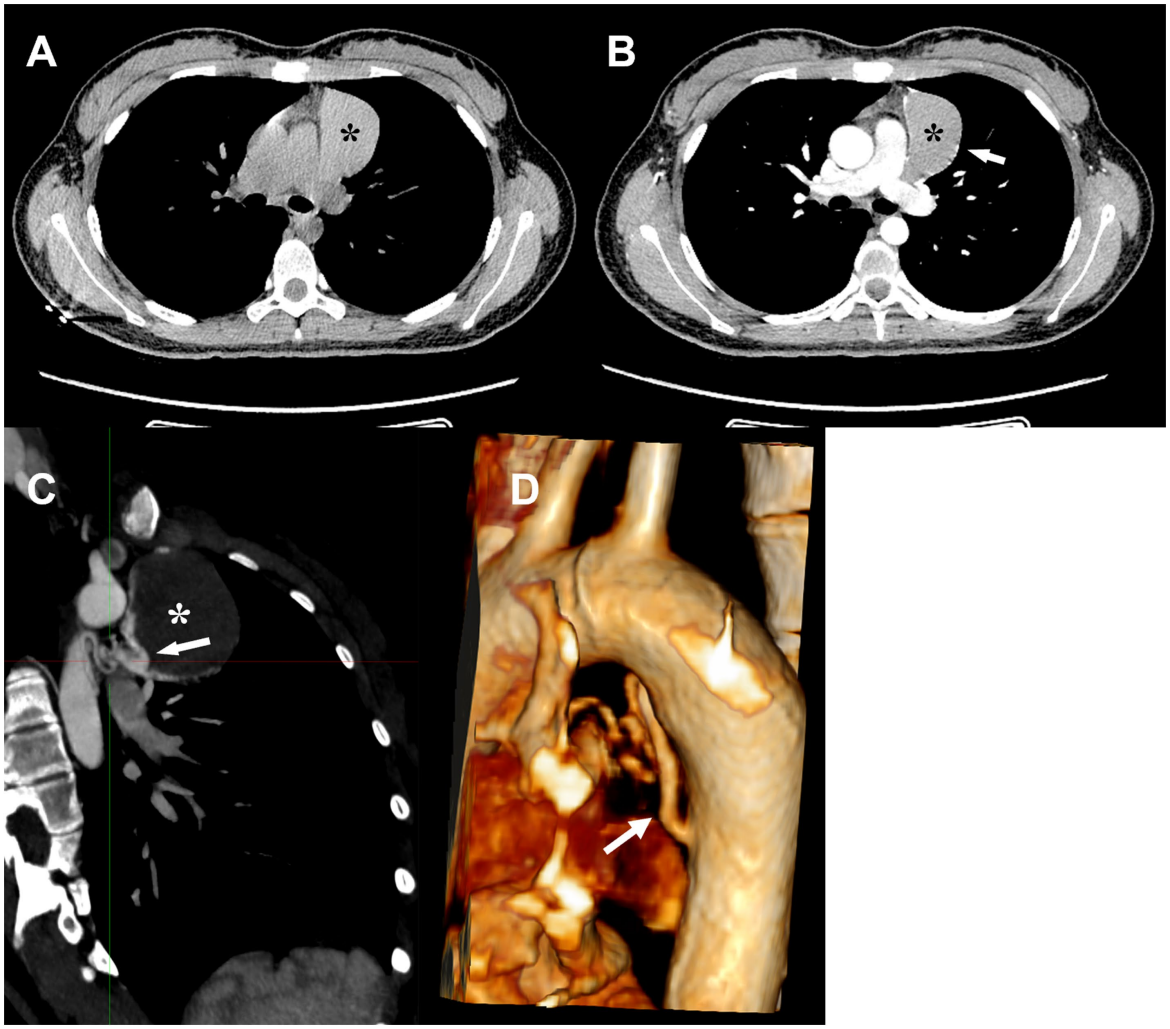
Compared with the CT scan without contrast (A), the cyst wall was enhanced on contrast-enhanced images (B). Sagittal CT image (C) and 3D reconstruction image (D) demonstrated a feeding vessel originating from the descending aorta and supplying the cyst. Black and white *: cyst, white arrowhead: branch artery of the descending aorta.
